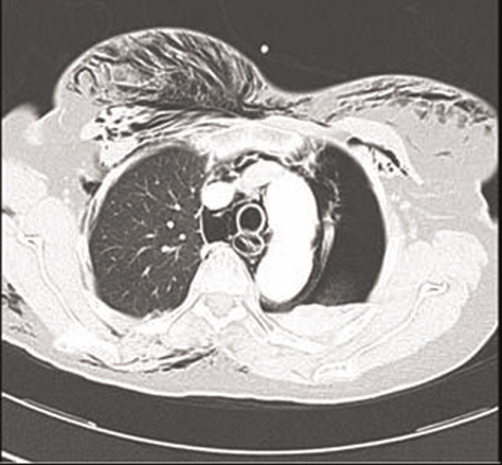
CT study showing extensive subcutaneous emphysema and left-sided pneumothorax, but no demonstration of the esophageal defect.
